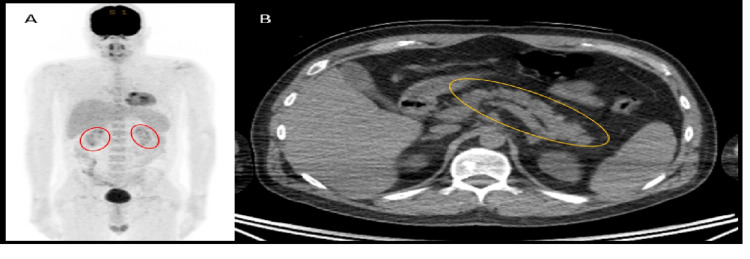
Normal PET-CT planar image six months post COVID recovery showing kidneys outlined by red oval shapes, and no abnormal FDG uptake centrally in the region of the pancreas. B. The pancreas (orange oval) has a normal morphology on CT with no calcification to suggest chronic pancreatitis PET: positron emission tomography; FDG: 18-fluoro-2-deoxyglucose
